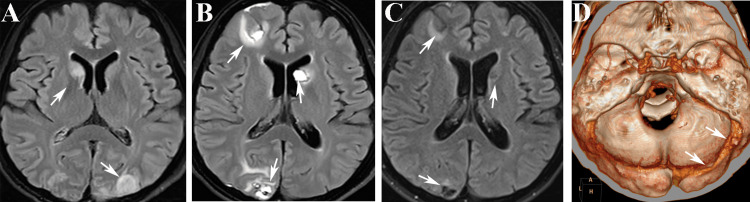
The MRI T2 flair images and cervical CTV obtained during the course of the illness A shows the MRI image taken during the first seizure episode while hospitalized in the orthopedic department (white arrows indicate ischemic lesions in the right caudate nucleus head and left occipital lobe). B displays the MRI findings two weeks after the hemorrhage (white arrows indicate ischemic lesions with necrosis in the right frontal lobe, occipital lobe, and left thalamus). C presents the follow-up cranial MRI conducted one month later (white arrows indicate old hemorrhagic lesions). D exhibits the three-dimensional reconstruction image of the cervical CTA (white arrows indicate two filling defects in the right transverse sinus). CTV, CT venogram
